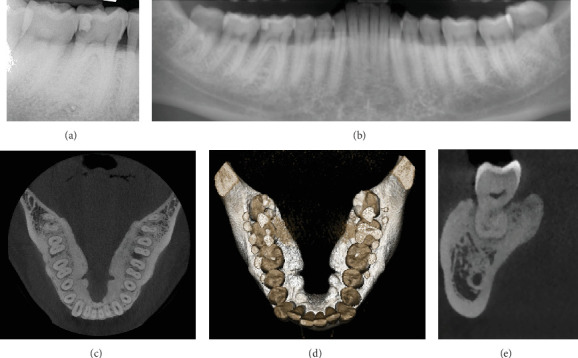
(a–e) Radiographic evaluation of the mandibular torus through various imaging modalities. (a) Periapical radiograph. (b) Panoramic radiograph (orthopantomogram). (c–e) CBCT scans revealing the detailed three-dimensional structure and extent of the torus.
